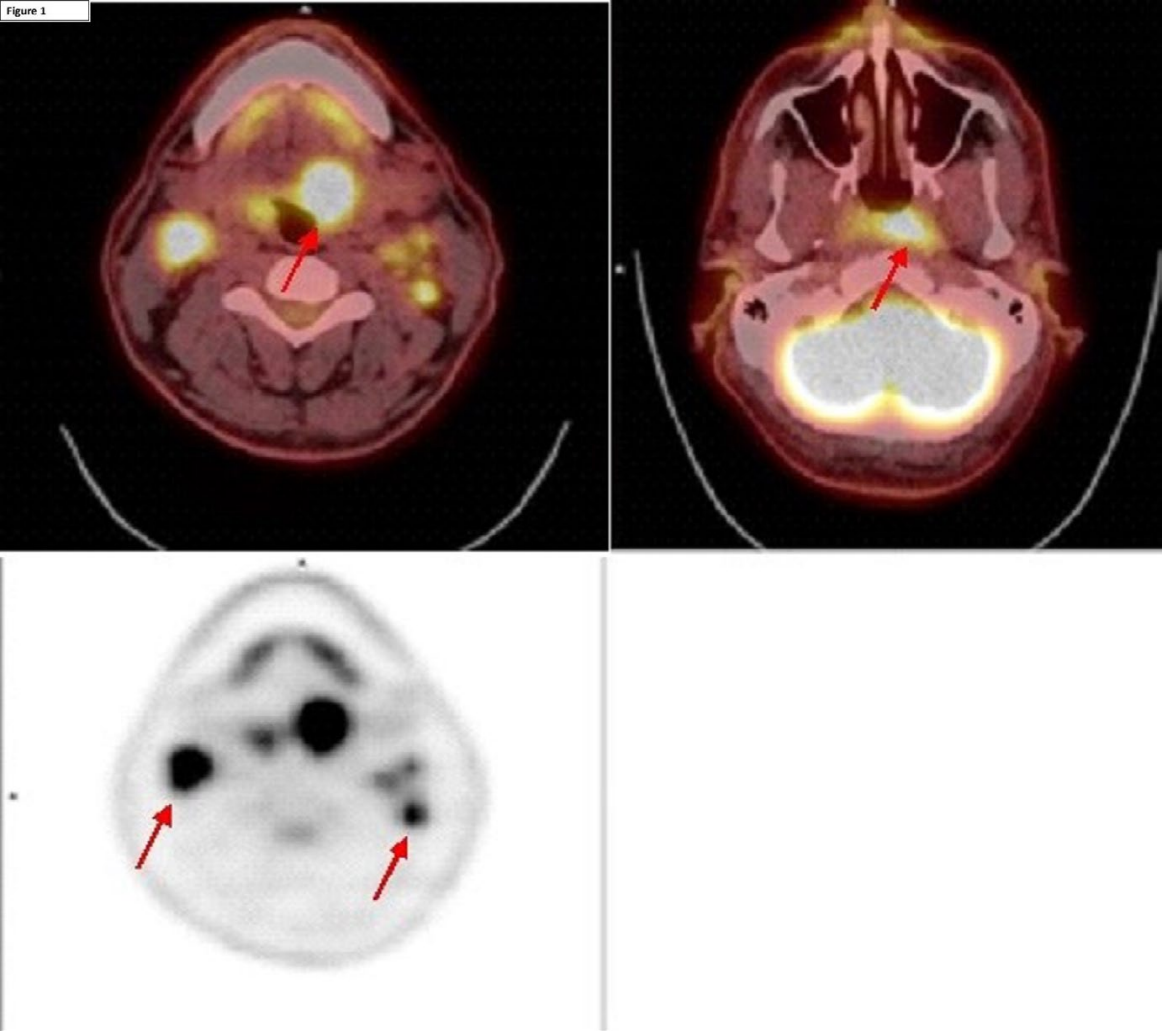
Axial section PET CT (top left), intense FDG uptake within tongue base mass (top right) higher FDG uptake on the left side of nasopharynx (bottom), and bilateral FDG avid cervical nodal metastases.
